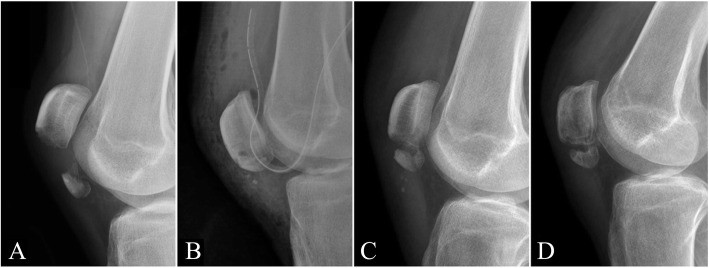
A man aged 33 years underwent transosseous reattachment for right patella inferior pole fracture. Knee radiographs A preoperation, B immediately after surgery, C 3 months postoperation, and D 9 months postoperation. Although postoperative radiological loss of reduction and nonunion were noted 3 months after surgery, patella height was maintained, and no further displacement was observed
